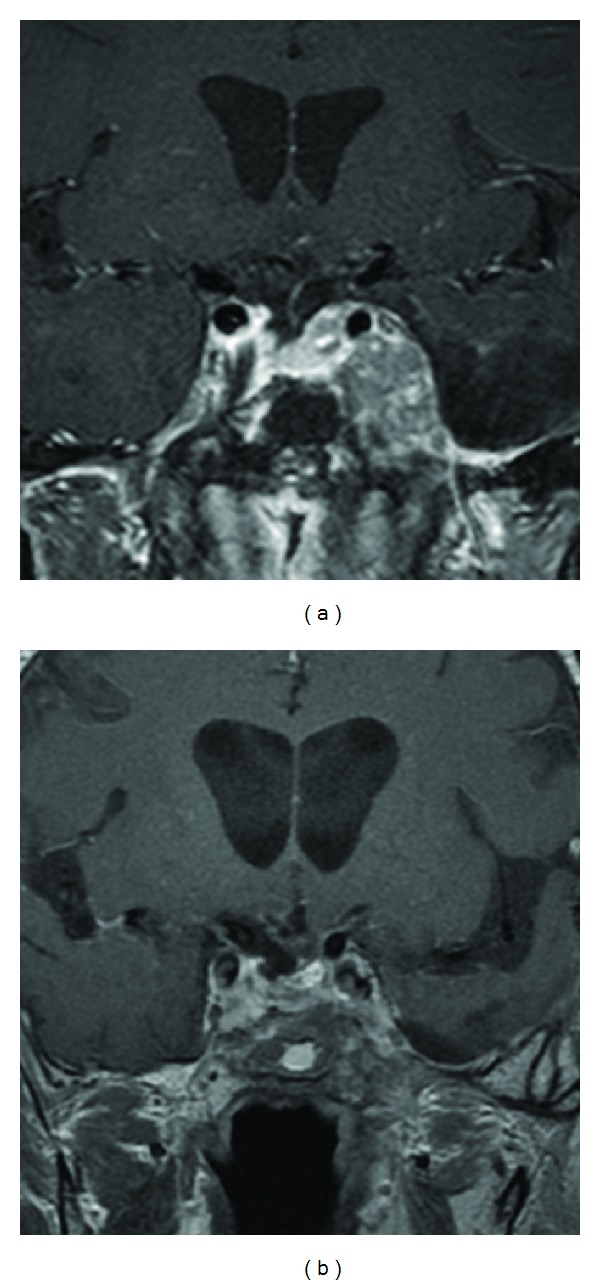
Pituitary T1-weighted postgadolinium coronal MRIs before (a) and after (b) 3 cycles of cisplatin and etoposide demonstrating significant tumor shrinkage of residual left cavernous sinus and Meckel's cave carcinoma.
